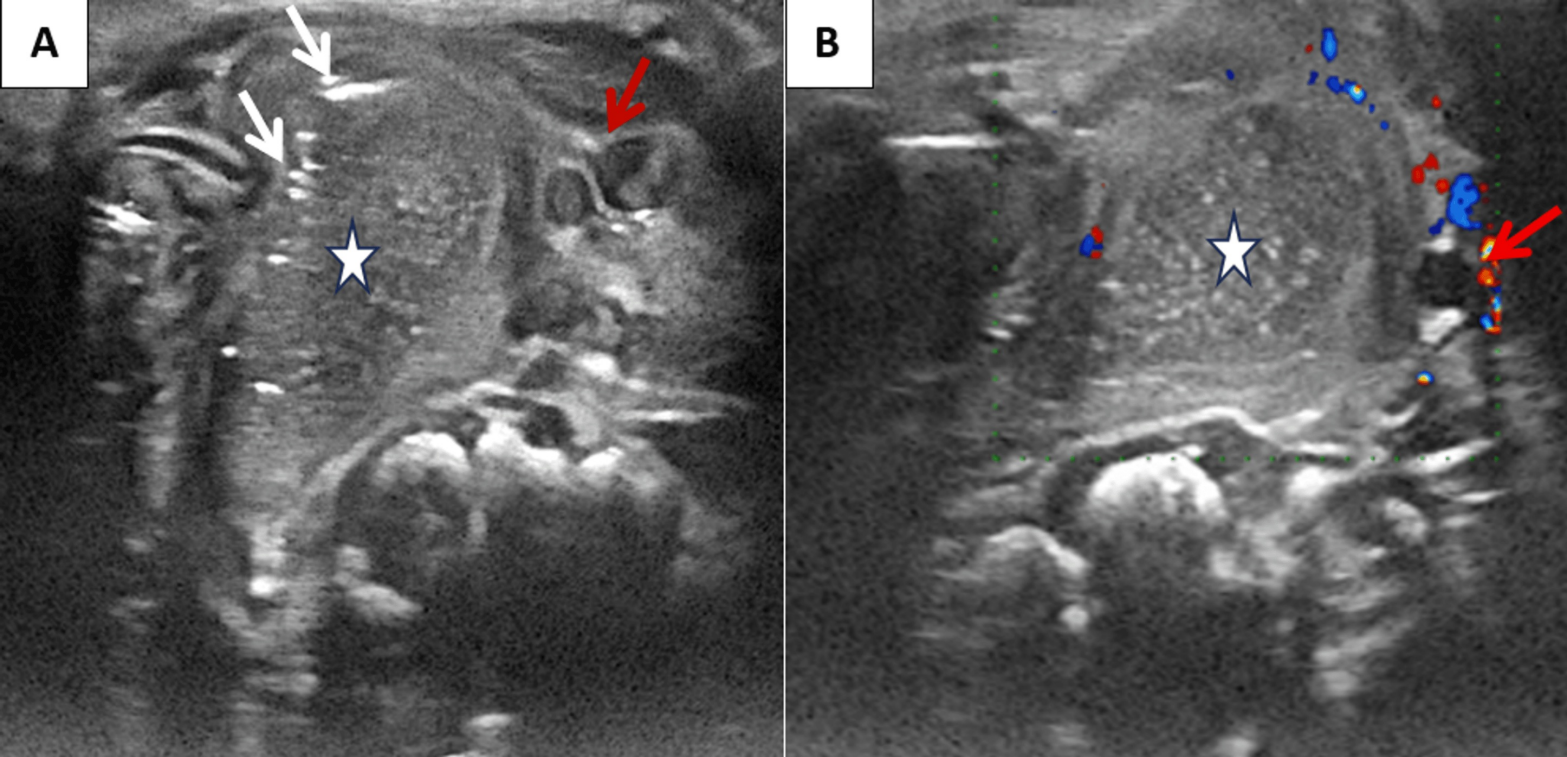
Ultrasound images in axial sections The images before (A) and after (B) color Doppler show a right lateral cervical mass (star) with fluid content generating a posterior acoustic shadow and containing hyperechoic foci consistent with air content (white arrow), showing no color Doppler uptake, and displacing the jugulocarotid axis laterally (red arrow).
